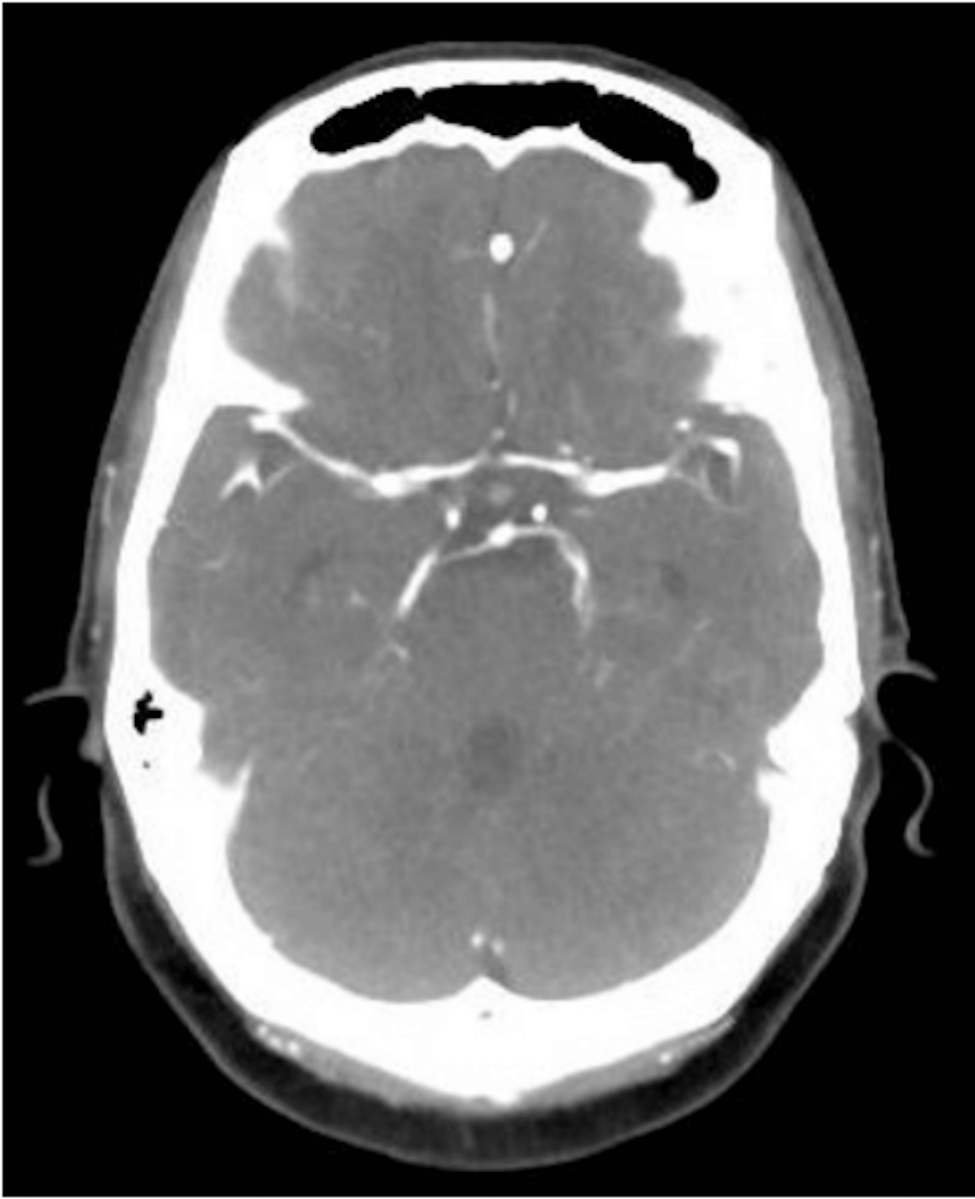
CT angiography Brain with no significant findings
